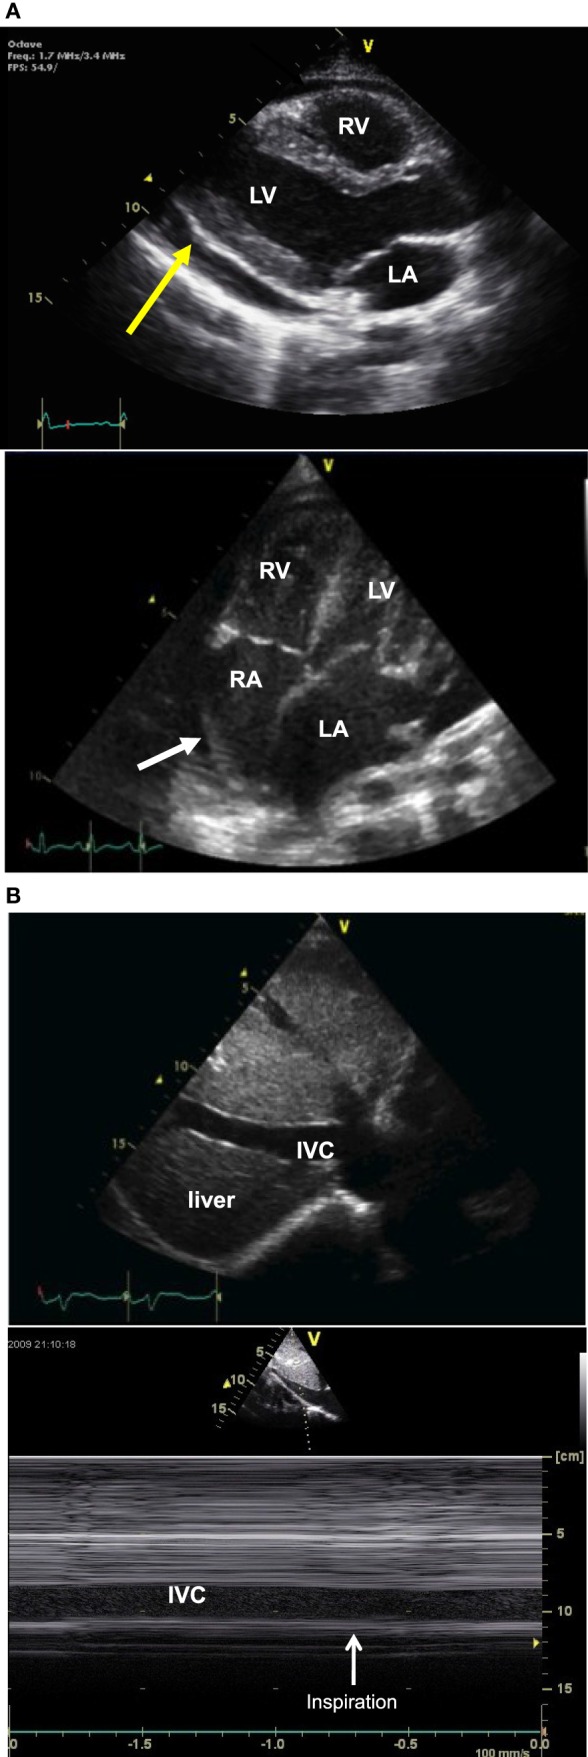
Two-dimensional (2D) echocardiography picture (A) obtained from a parasternal long axis view (picture above) showing an echo-free space (yellow arrow) between the two pericardial layer typical of pericardial effusion, and picture obtained from an apical four-chamber view (picture below) showing right atrial free wall collapse (white arrow) consistent with cardiac tamponade. 2D echo picture [(B), above] obtained from a longitudinal subcostal view showing a dilated IVC and M-mode echocardiography [(B) below] showing no respiratory variation of IVC size. IVC, inferior vena cava; LA, left atrium; LV, left ventricle; RA, right atrium; RV, right ventricle.
